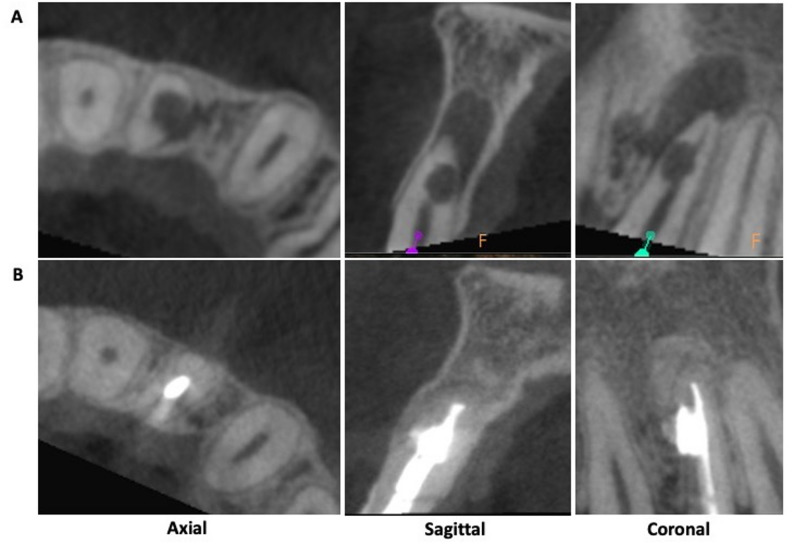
Cone-beam computed tomography (CBCT) evaluation of the perforating internal root resorption lesion and long-term healing outcome. A Preoperative CBCT views in axial, sagittal, and coronal planes demonstrating a well-defined resorptive cavity originating from the pulp space and extending toward the external root surface, confirming perforation of the root wall of tooth #7. Three-dimensional imaging allowed precise localization of the defect and assessment of its relationship to the surrounding periodontal structures. A periapical bone defect associated with the same tooth is also evident. B CBCT images obtained at the eight-year follow-up in axial, sagittal, and coronal planes demonstrating complete osseous healing, re-establishment of cortical bone continuity, and stable repair of the resorptive defect without evidence of recurrent resorption or periapical pathology
